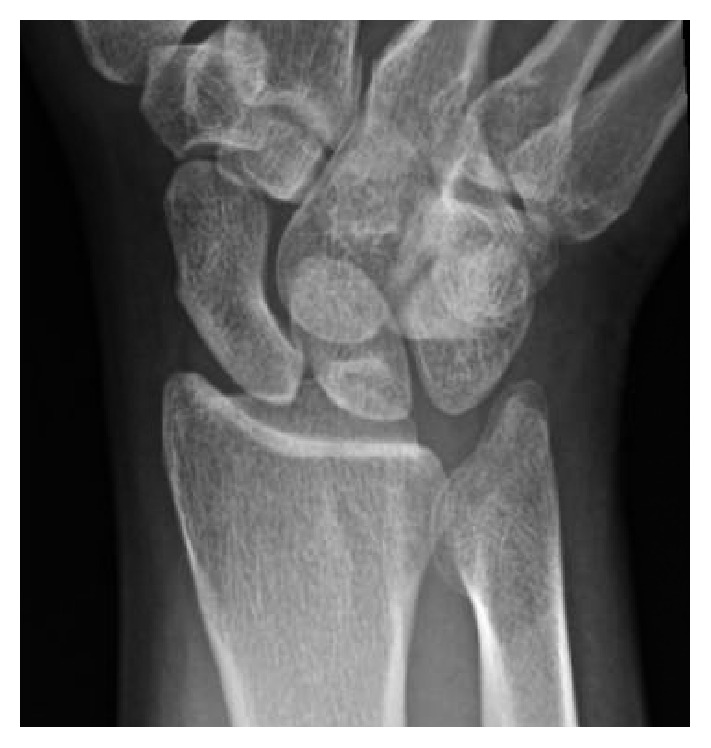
A scaphoid view obtained 6 weeks after presentation without radiographic evidence of a scaphoid waist fracture.
